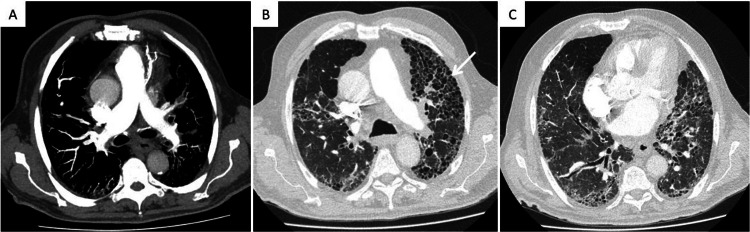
Chest computed tomography angiography excluding pulmonary embolism (A). Parenchymal changes in relation to fibrotic hypersensitivity pneumonitis (B, C), with a honeycomb pattern (white arrow)
